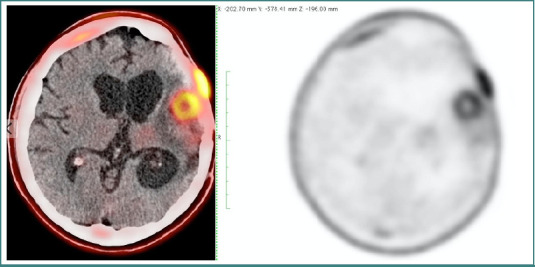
Pet CT scan FDG. Hypermetabolic temporal lesion compatible with residual or recurrent lesion.
